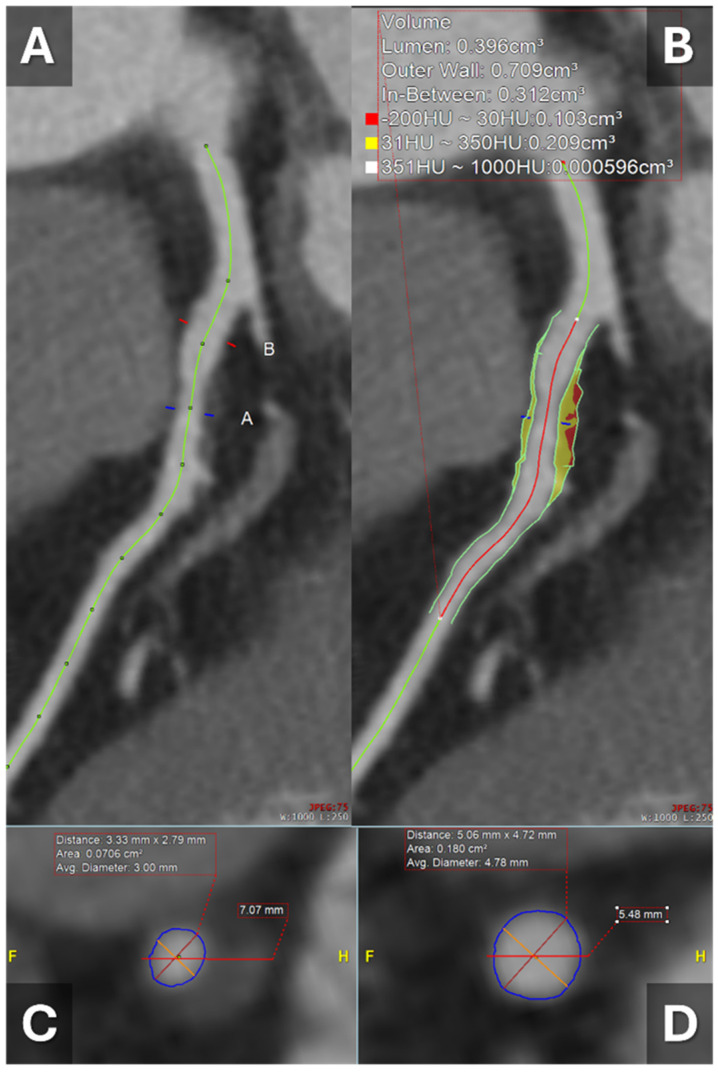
A 61-year-old male presented with atypical chest pain and proceeds to CCTA. He had moderate non-obstructive coronary atherosclerosis, which did not require revascularization; however, based on these findings, the patient was commenced on statin therapy and intensive risk factor modification. (A) Curve multiplanar reconstruction of the LAD demonstrating non-obstructive low-attenuation plaque at the level of “A” with luminal narrowing compared to level “B”. (B) Plaque analysis of the LAD stenosis showing low Hounsfield units, suggesting soft high-risk plaque with positive remodeling. (C) Cross-sectional imaging showing moderate stenosis at LAD level “A”. (D) Cross-sectional imaging showing no stenosis at LAD level “B”. Abbreviations: CCTA = coronary computed tomography angiography, LAD = left anterior descending artery.
